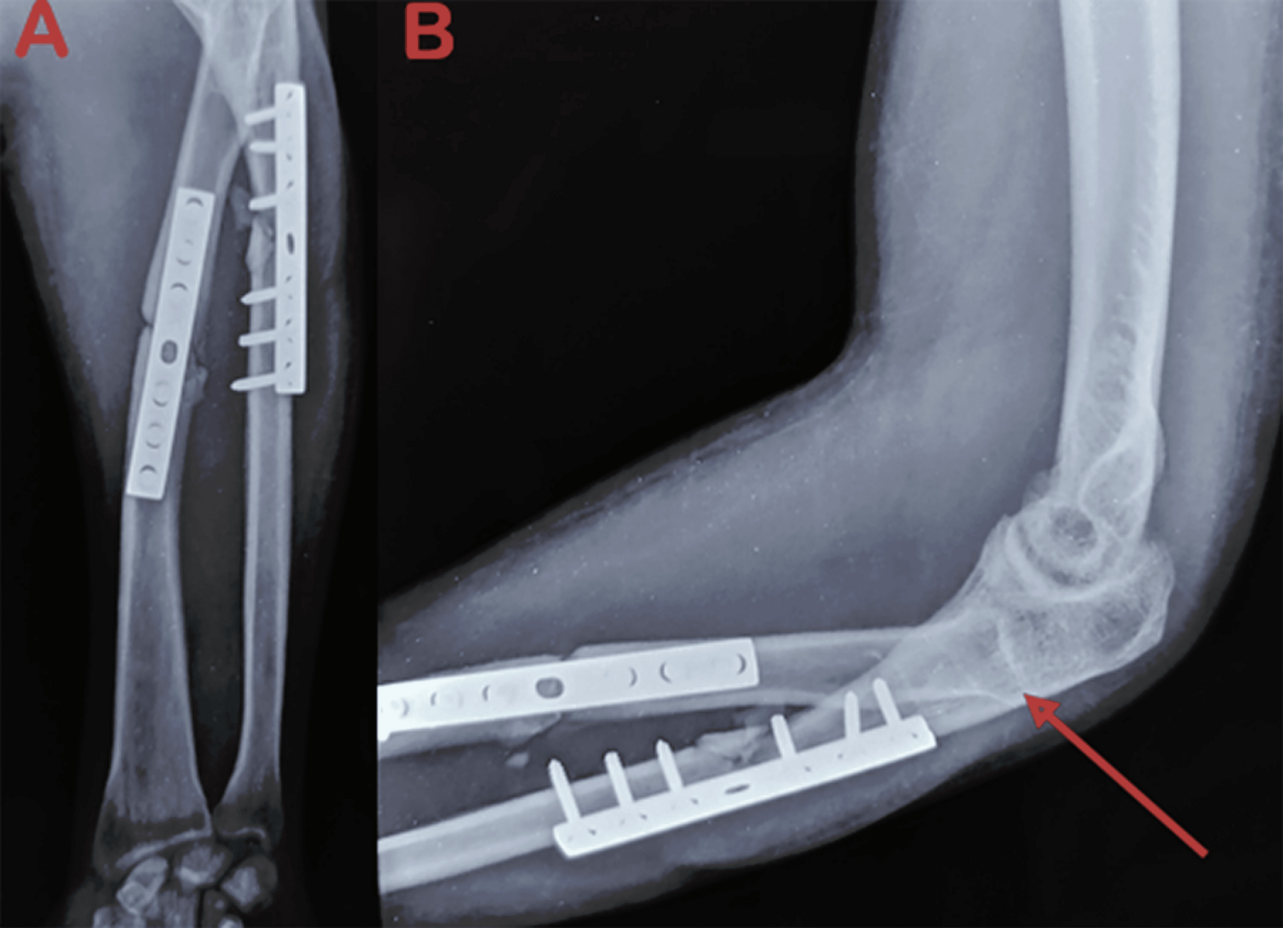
Radiographic view of the left elbow and forearm (A) Anteroposterior view of the left forearm showing a well-aligned ulnar and radial shaft. (B) Lateral view of the elbow and forearm showing a posterior dislocation of the radial head.
